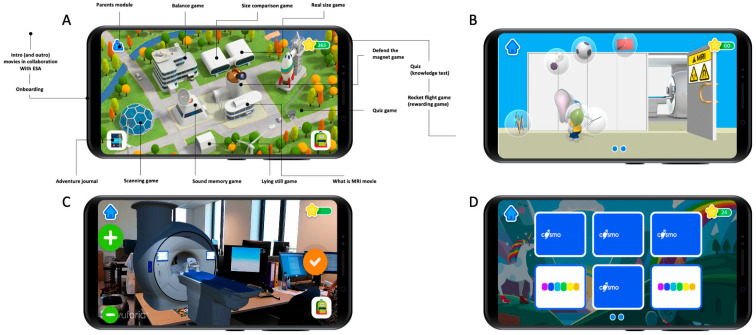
App design and look. (A) Overview of the space campus with all the buildings and minigames. (B) Screenshot of the “Defend the magnet” minigame, where children have to prevent metal objects from flying into the MRI room by tapping on them. (C) “Real size game”. Using augmented reality, children can project the MRI scanner in their room and scale it until they think it is “real-sized”. (D) “Sound memory game” minigame, where children need to pair the same MRI sounds by simultaneously turning two identical sound cards.
